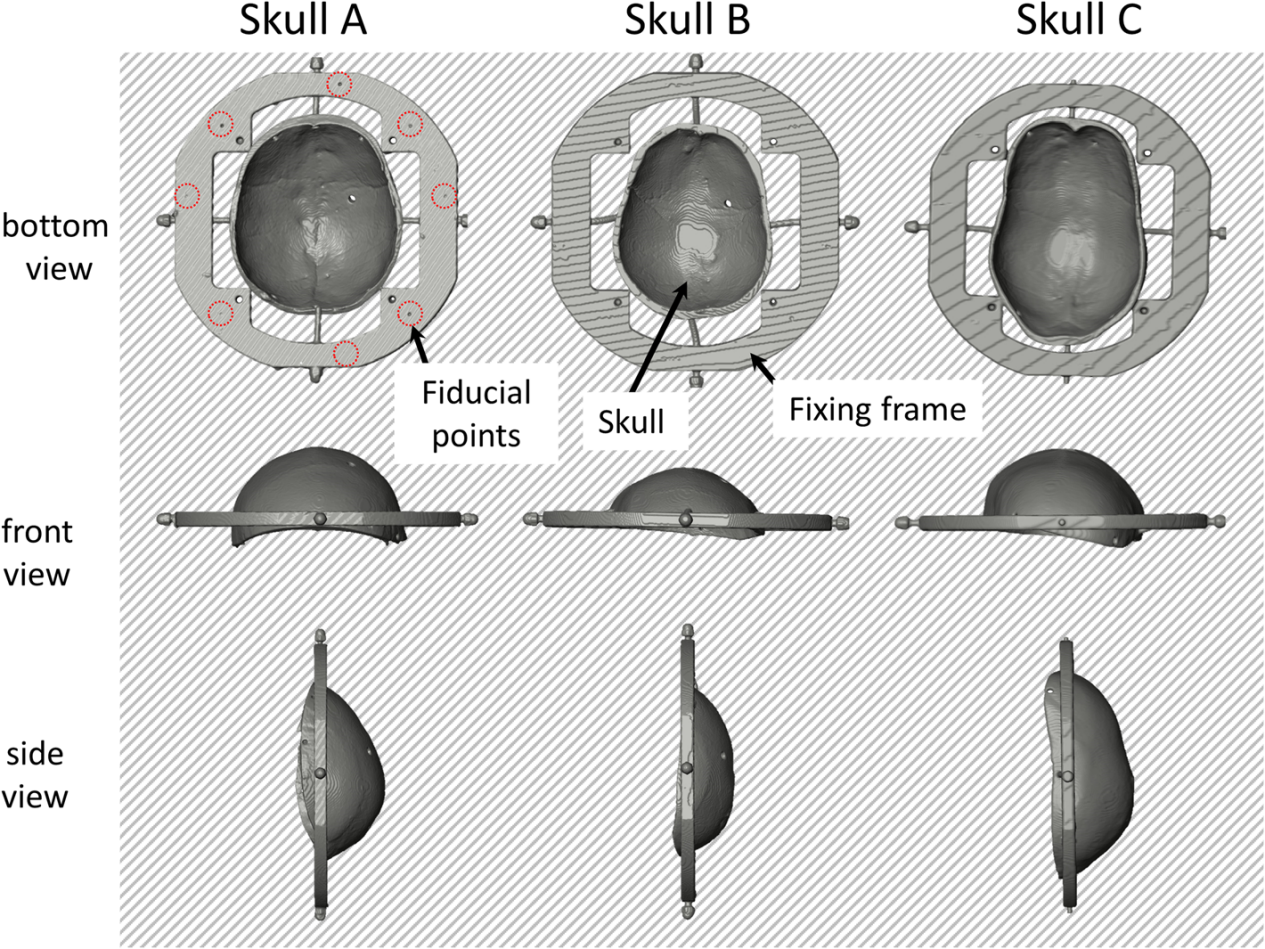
Reconstructed volume rendering of three skulls with their fixing frame. The volume of skull and fixing frame were reconstructed from CT volume images. The bottom, front and side view of three skull volume were shown in the figure. The visible fiducial points of skull A (seven points) were marked using red-doted circles. The variation of shape and surface curvature of each skull could be observed
